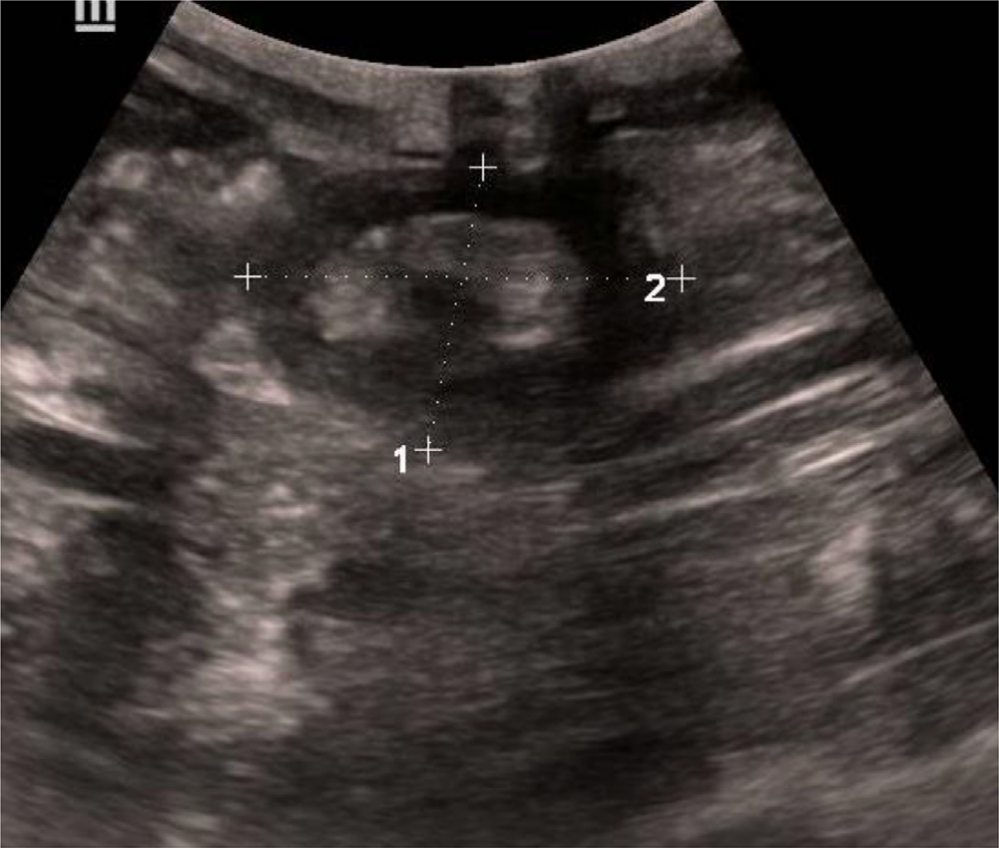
Ultrasound image showing the characteristic ``sandwich'' appearance of intestinal intussusception.
